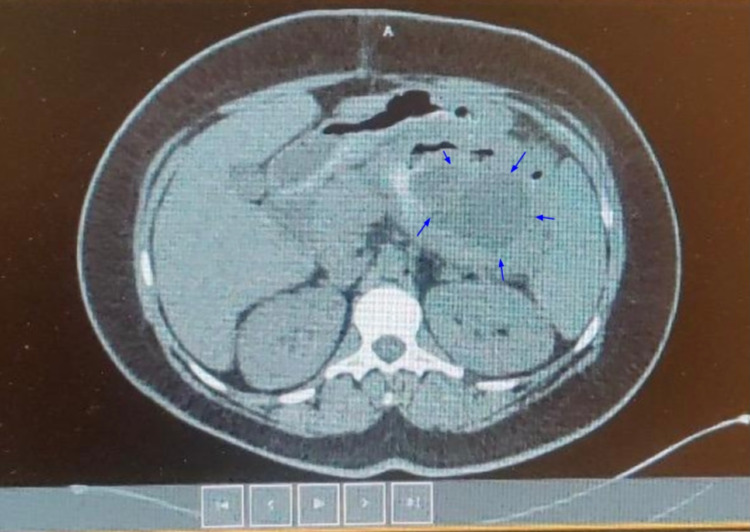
Non-contrast CT scan of the abdomen three weeks post-surgery. Blue arrows highlighting the margins of the pancreatic cyst
